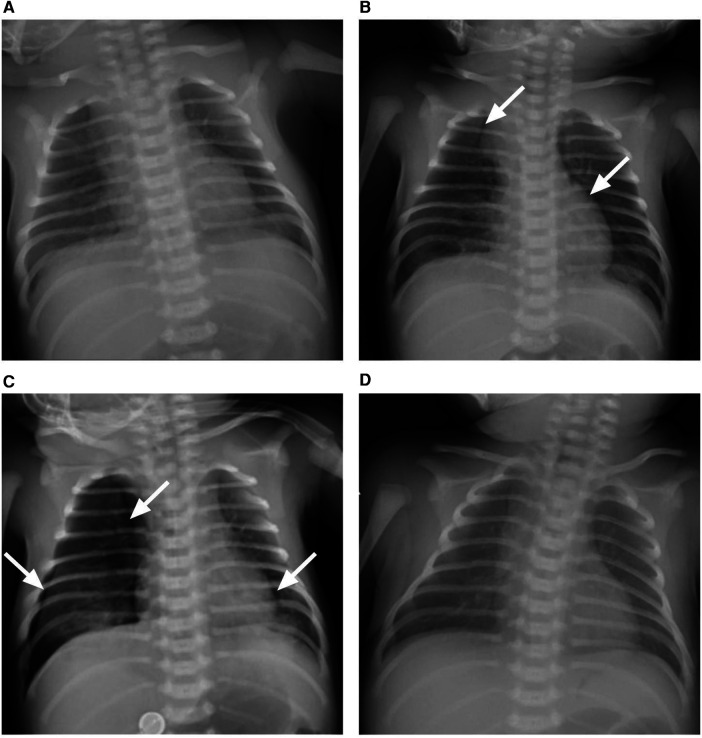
The chest x-ray presentation of the patient. (A) Bilateral pulmonary exudative lesions were found on admission, revealing pneumonia. (B) Lucency shadows were observed at two sides of para-mediastinum as marked with white arrows on day 2 after admission, suggesting pneumothorax. (C) Pneumothorax deteriorated, and the right lung was remarkably compressed as marked with white arrows on day 3 after admission; (D) bilateral pulmonary exudative lesions and pneumothorax were absorbed on day 10 after admission.
